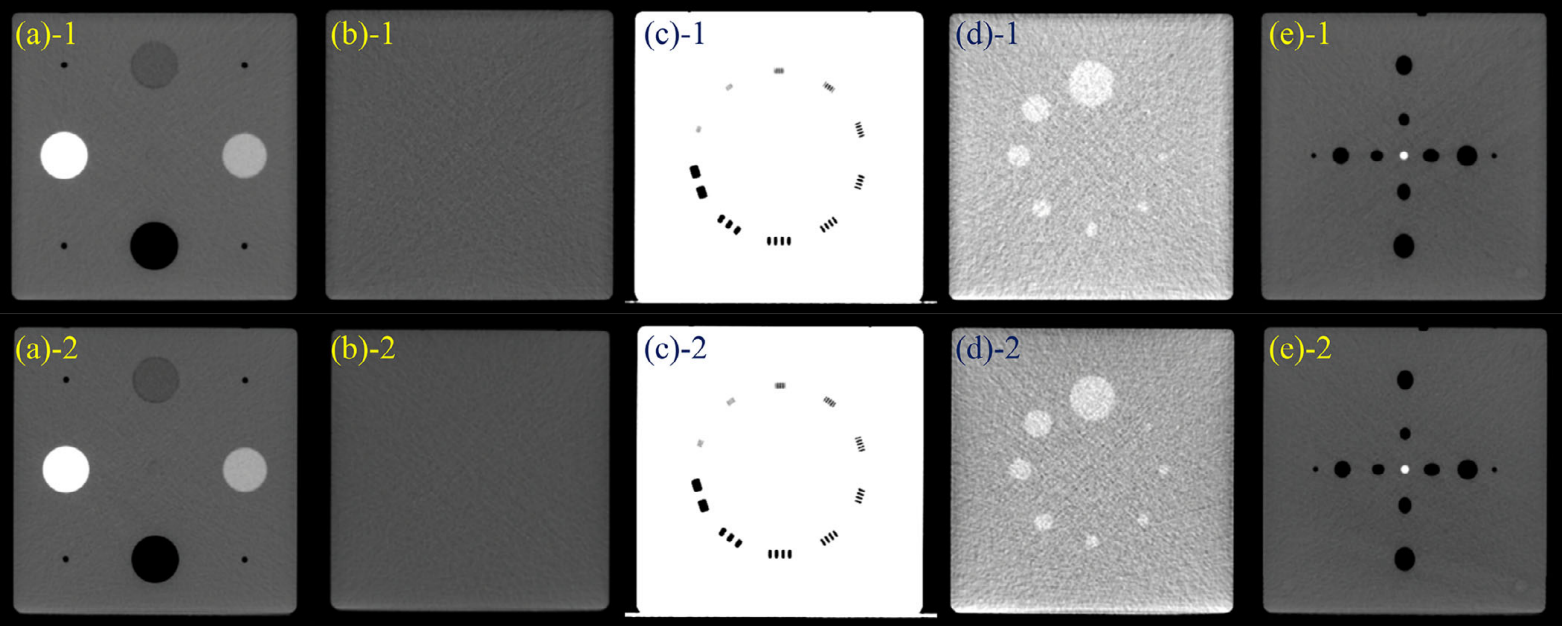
Transverse CT images from (1) Big Bore RT and (2) Discovery RT Gen3: (a) HU constancy, (b) uniformity, (c) spatial resolution, (d) low contrast, (e) coincidence check.
